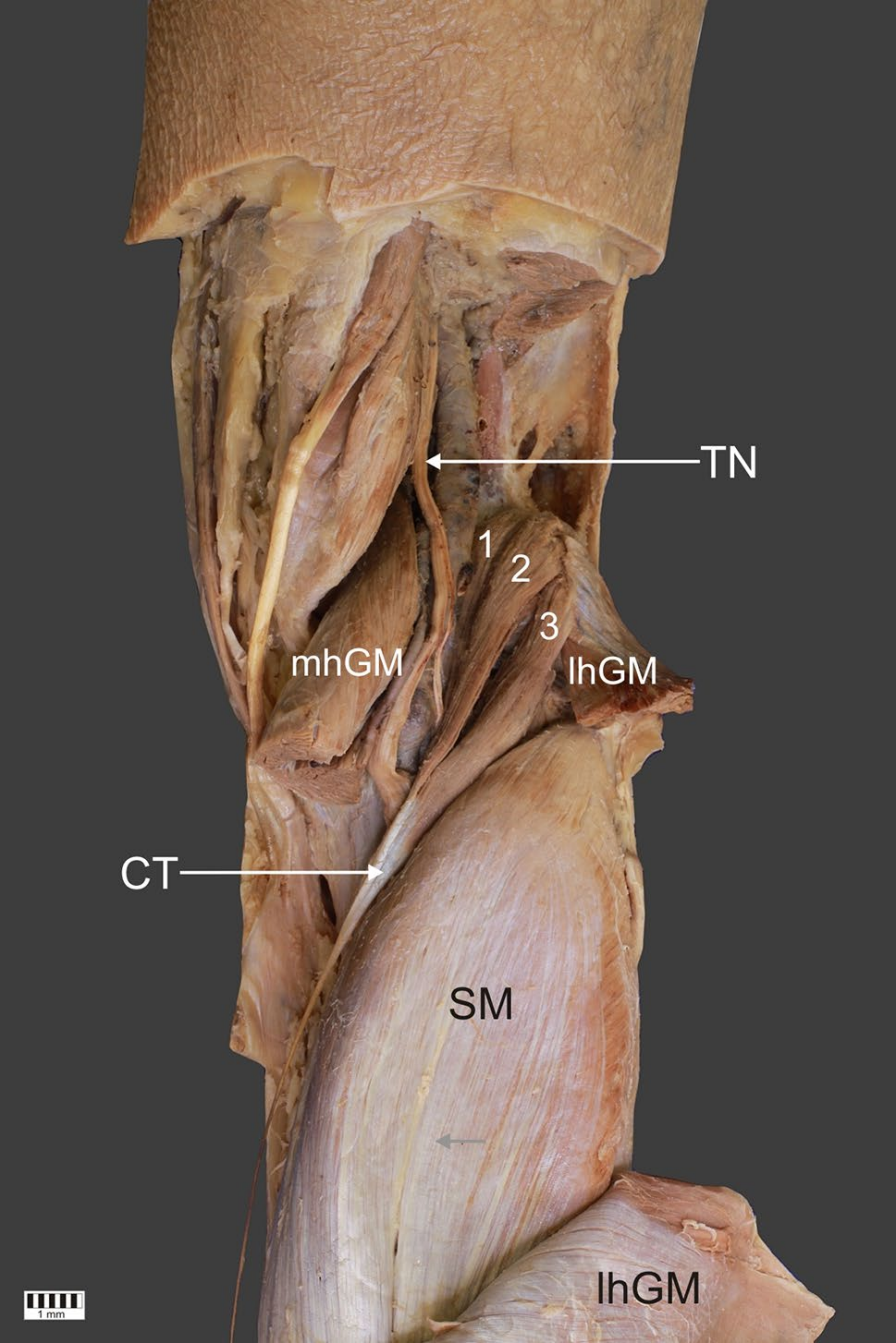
A rare three-headed plantaris muscle. *1* first head of the plantaris muscle, *2* s head of the plantaris muscle, *3* third head of the plantaris muscle, *TN* tibial nerve, *lhGM* lateral head of the gastrocnemius muscle, *mhGM* medial head of the gastrocnemius muscle, *CT* common tendon, *SM* soleus muscle
